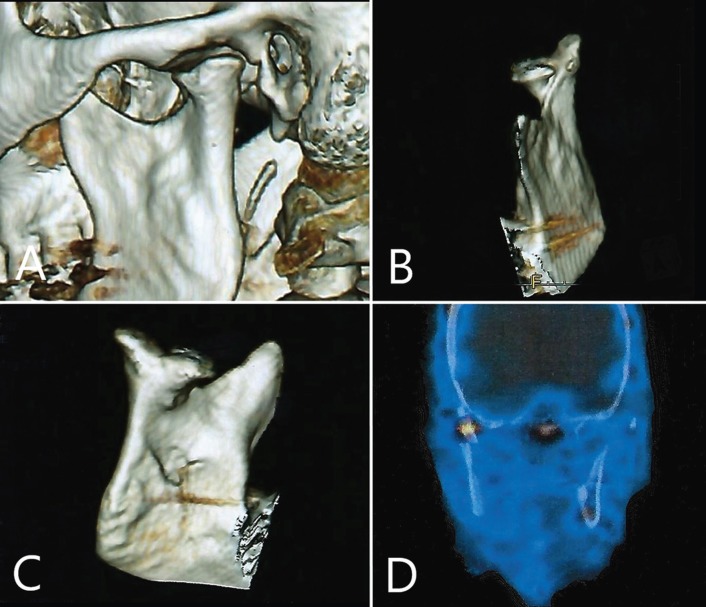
Case 2. Computed tomography 3D reconstruction. A) Sagittal view. B) Anterolateral view. C) Posteromedial view. D) SPECT frontal view.
